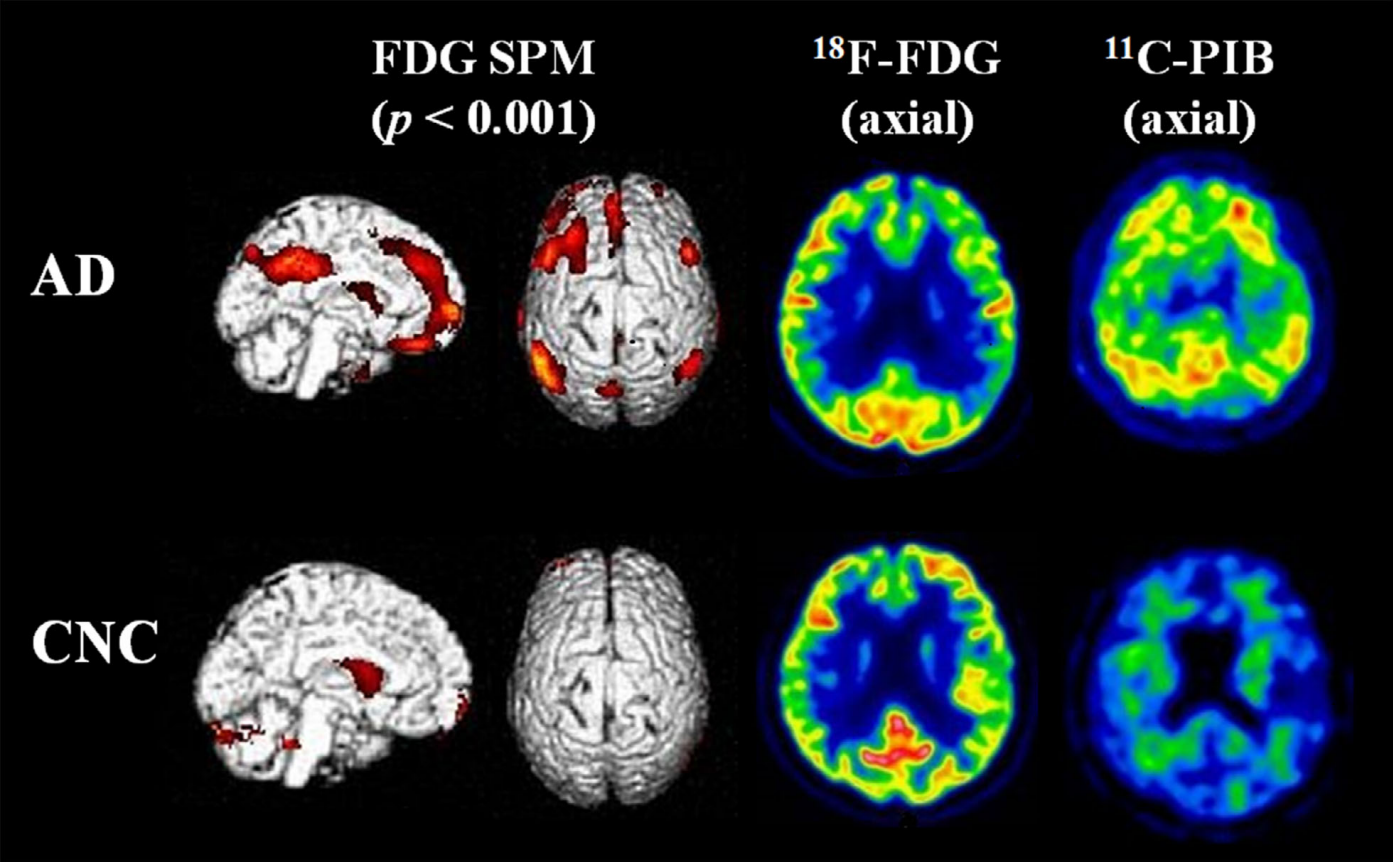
Representative FDG and PiB PET patterns in patients with AD and NCs. The left two rows show SPM T‐maps (*P* < 0.001) rendered on the ch2bet template brain. The right two rows display axial images of FDG PET and PiB PET, respectively. Hypometabolism in inferior parietal lobule, precuneus/posterior cingulate gyrus, bilateral dorsolateral frontal lobe, and medial prefrontal cortex on FDG PET and amyloid positivity on PiB PET are seen in a patient with AD. Normal glucose metabolism on FDG PET and amyloid negativity on PiB PET from a healthy individual are also presented. Abbreviations: FDG, fluorodeoxyglucose; SPM, statistical parametric mapping; PiB, Pittsburgh Compound‐B; PET, positron emission tomography; AD, Alzheimer’s disease; NC, normal control.
